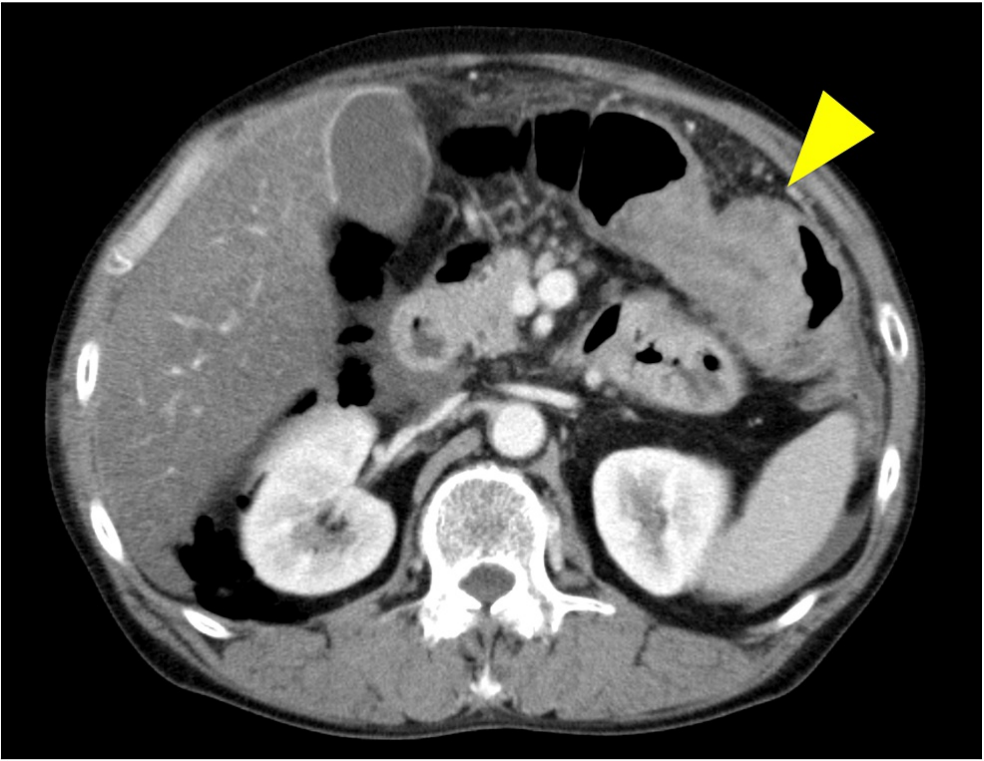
Contrast-enhanced abdominal CT Contrast-enhanced CT revealed perforation of the cecum and the intussusception of a 50 mm tumor in the transverse colon (yellow arrowhead).
